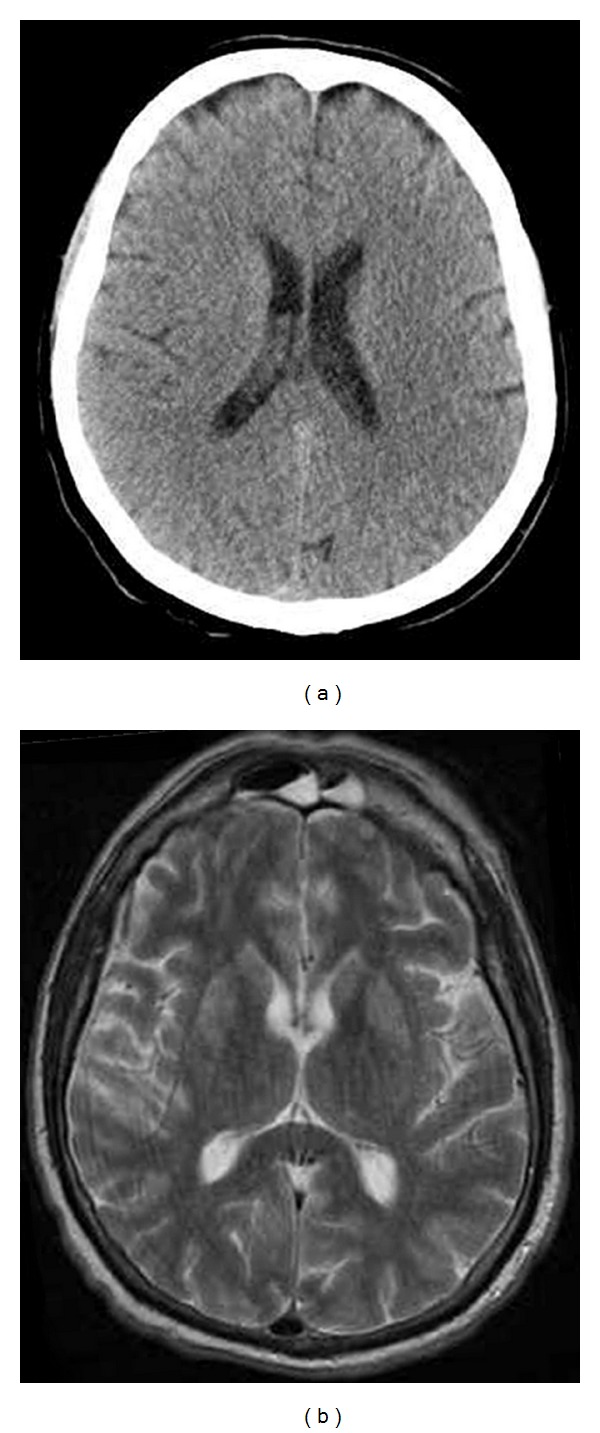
(a) An urgent cranial computed tomography scan was negative for cerebral oedema, hemorrhage, and structural lesions. (b) The magnetic resonance imaging, obtained three weeks after the initial event, demonstrated a mild hyperintensity in the lenticular nuclei and periventricular areas bilaterally in T2 sequences.
